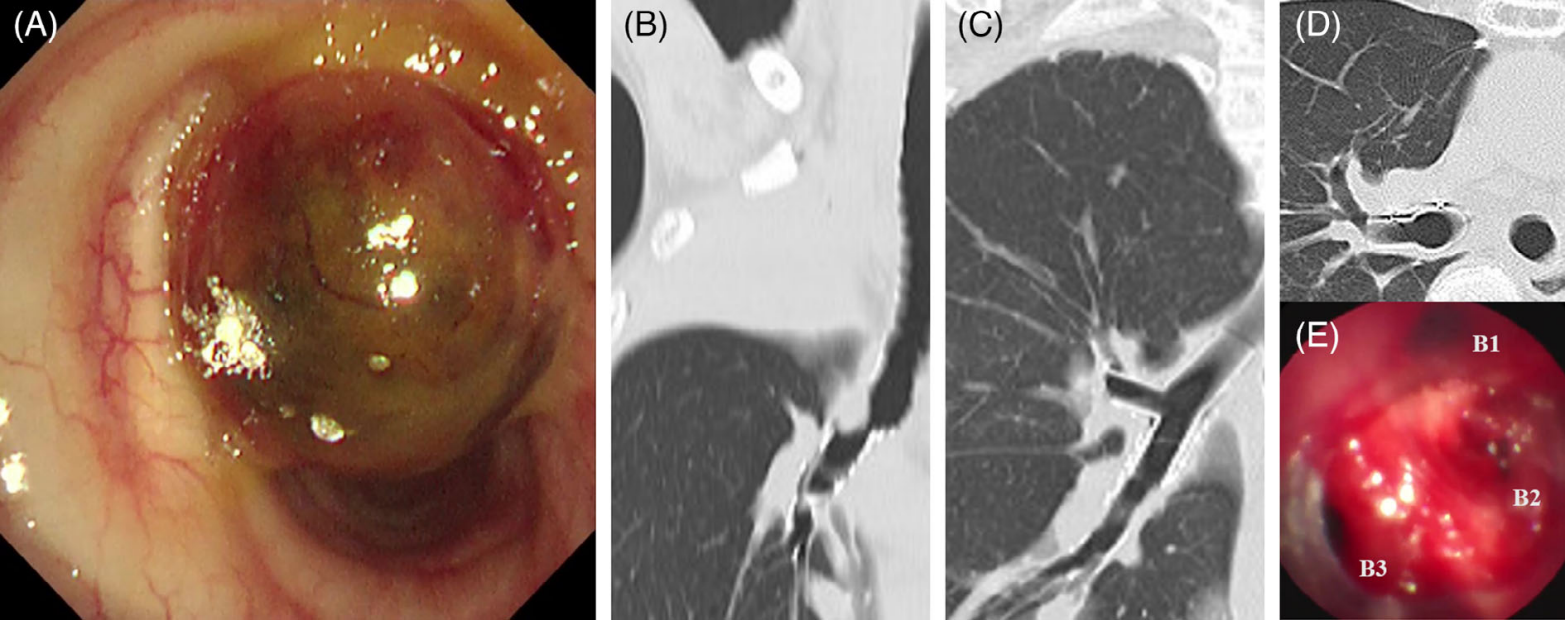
(A) Bronchoscopy revealed a large, red, smooth surface lesion with irregular vascularization and necrosis. (B) Chest computed tomography showed a lesion in the right main bronchus and right upper lobe atelectasis. (C, D) Chest computed tomography indicated that the OKI‐stent™ (the outer diameter and length of limbs for the right main stem bronchus, bronchus intermedius, and right upper lobe bronchus were 13–10–9 and 30–20–10 mm) was placed successfully in the right main bronchus and the upper lobe bronchus. The atelectasis was resolved. (E) The stent was extended near B2, where the tumour had originated, to avoid reinvasion.
